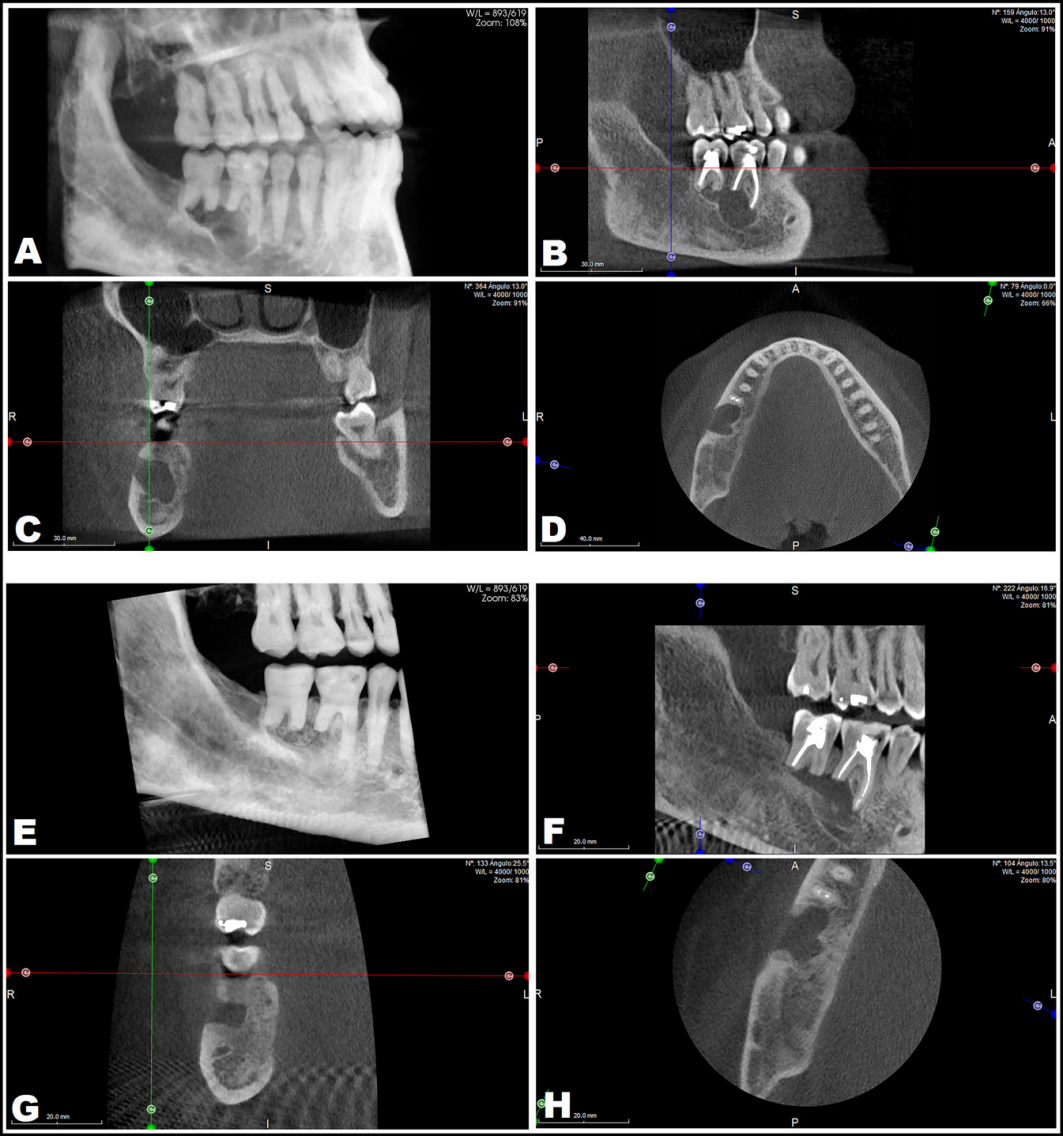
Cone-beam computed tomography scan. Situation before marsupialization. December 2019 (A-D). Situation 9 months after marsupialization. September 2020 (E-H).
